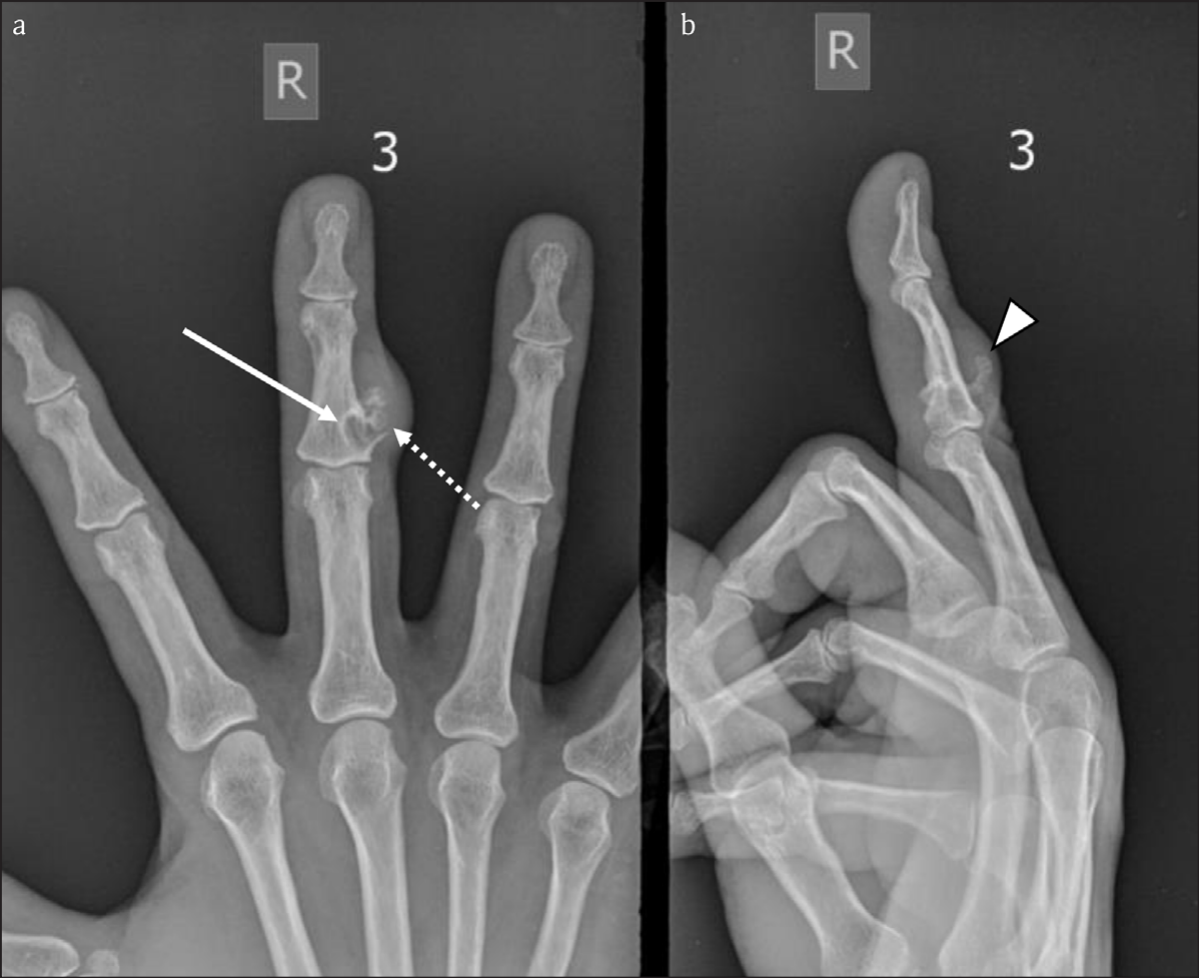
Case 2 – Conventional radiographs of the right third finger. AP (a) and lateral view (b). Note a juxta-cortical radiolucent lesion at the dorso-ulnar aspect of the middle phalanx. The smaller intramedullary part is well-delineated and surrounded by a rim of sclerosis (arrow in a). The larger exophytic component consists of a bony protuberance with adjacent soft tissue swelling (arrowhead in b). There is focal breakthrough of the cortex (dotted arrow in a).
